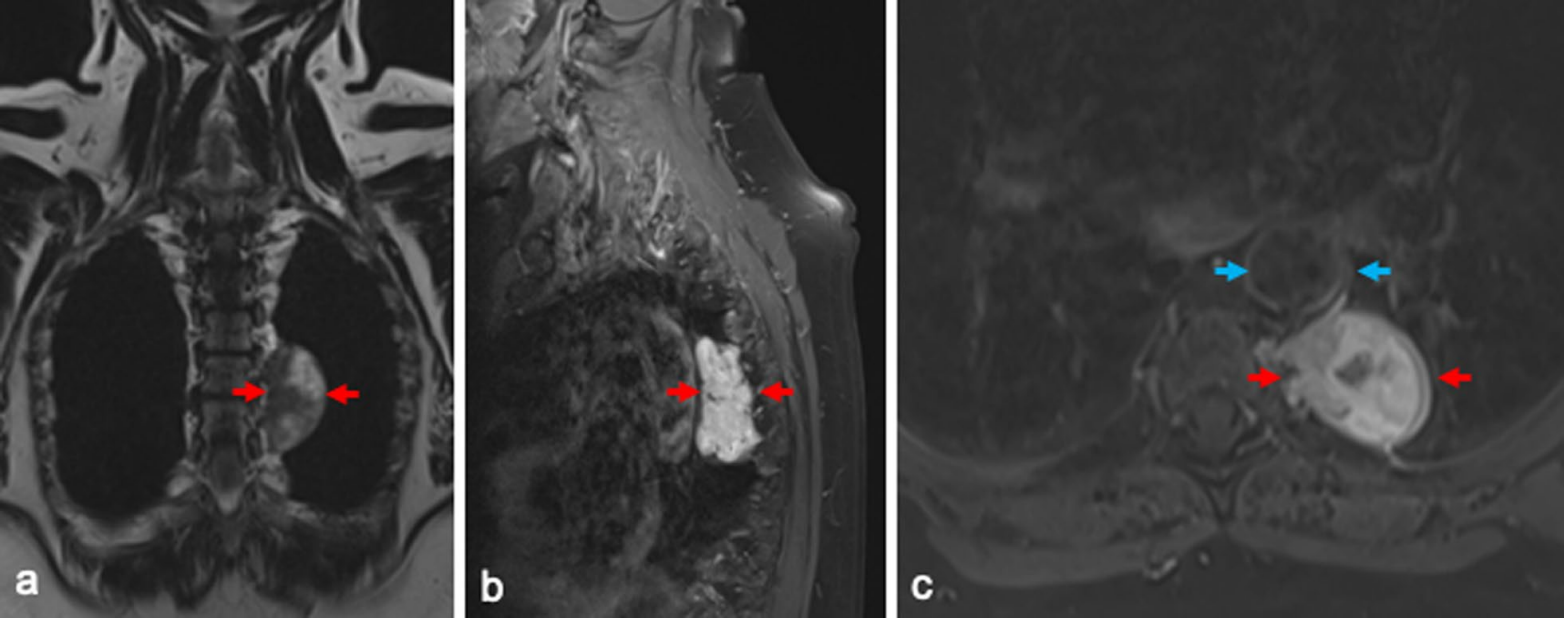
Thoracic magnetic resonance imaging showing the left paravertebral mass with a inhomogeneous signal on coronal T2-weigthed images, b high signal intensity on sagittal fat-saturated T2-weighted images, and c strong inhomogeneous enhancement on axial fat-saturated T1-weighted images. Red arrows show the tumor, adjacent to the aorta marked with blue arrows
